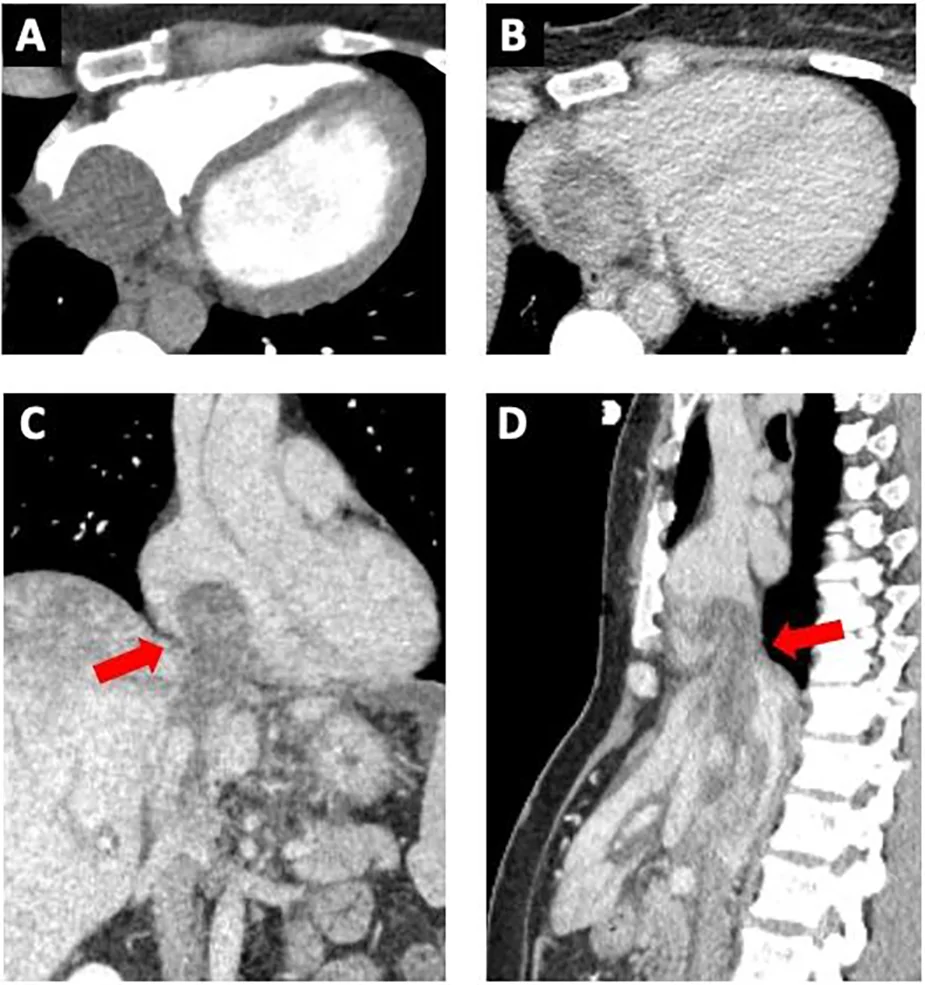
Contrast-enhanced computed tomography from different acquisitions demonstrating an inferior vena cava tumor with extension into the right atrium. (A) Pulmonary arterial phase in axial view, performed for suspected pulmonary embolism, showing a filling defect in the right atrium and initially suggesting a primary cardiac mass. (B) Axial view in venous phase demonstrating the tumor within the right atrium. (C) Coronal reconstruction in venous phase showing continuity of the tumor from the inferior vena cava into the right atrium (arrow). (D) Sagittal reconstruction confirming continuity of the tumor from the inferior vena cava into the right atrium (arrow), without evidence of regional metastases.
